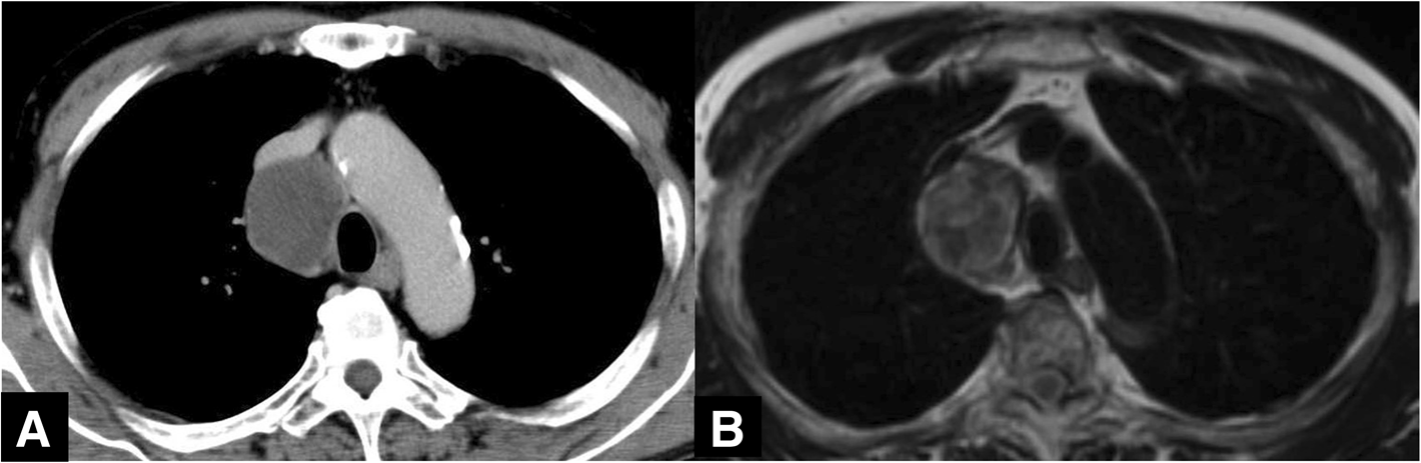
a Chest CT scan shows a low-density mass in the middle mediastinum surrounded by the brachiocephalic vein, brachiocephalic artery, and trachea. b T2-weighted MRI reveals the main tumor to have a heterogeneous isodense signal intensity, and the tumor was encapsulated by a low-signal area
